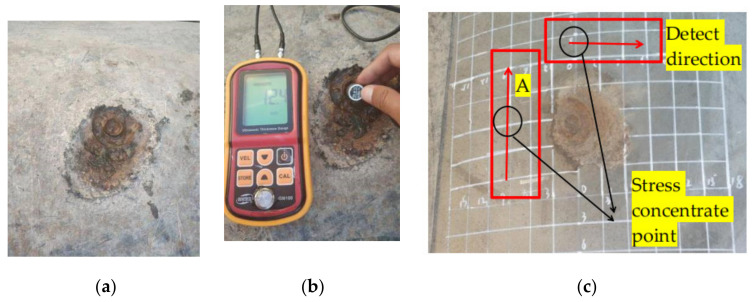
The excavation verification results: (a) Damage of anticorrosion coating; (b) Ultrasonic thickness measurement; (c) Pipe wall damage.
